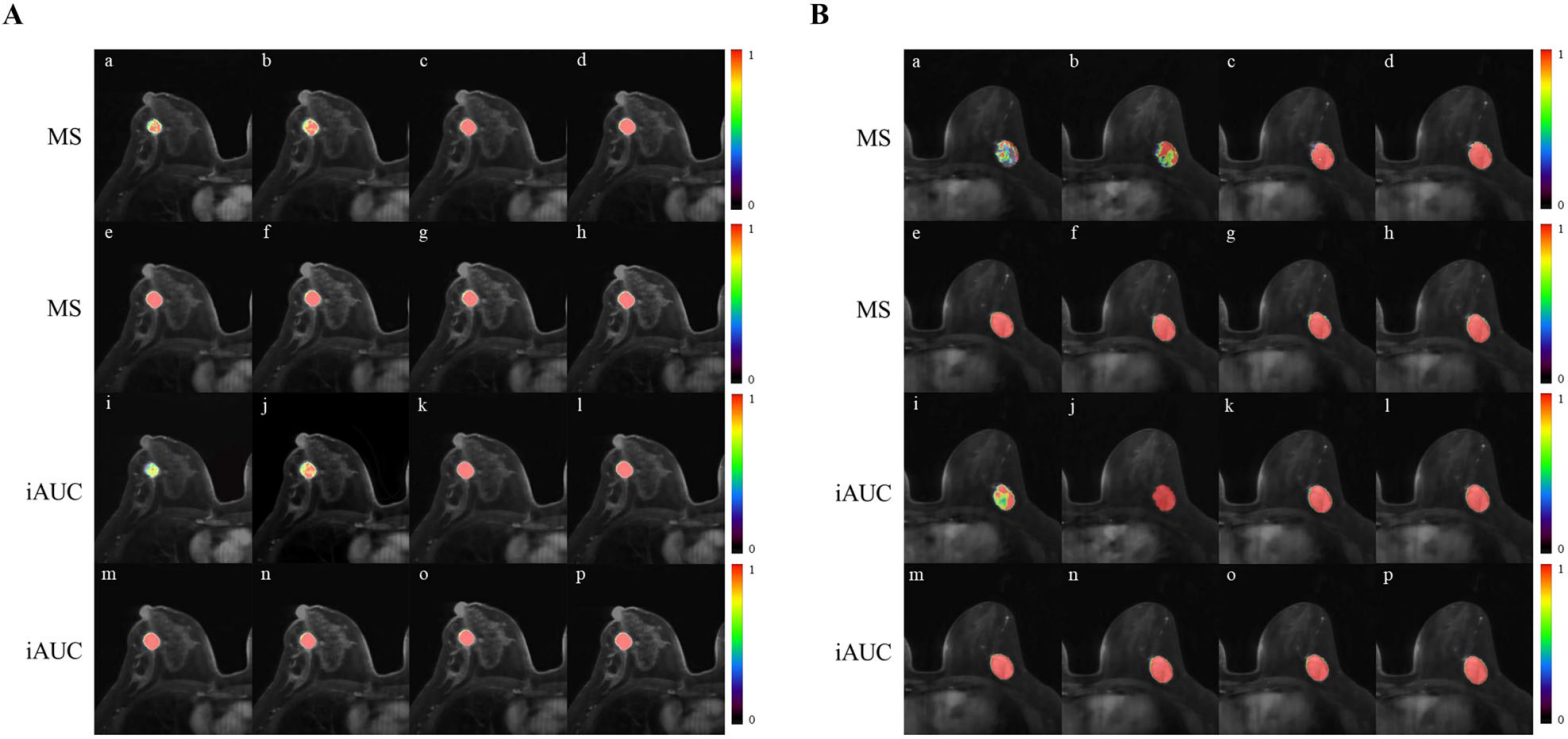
A Ultrafast breast DCE-MRI scans in a 49-year-old woman with pathologically confirmed benign fibroadenoma in the right breast. The first two rows (a–h) show color maps of the MS from SD_40.5s_ to SD_135s_. The last two rows (i–p) show color maps of iAUC from SD_40.5s_ to SD_135s_. B Ultrafast breast DCE-MRI scans in a 53-year-old woman with pathologically confirmed invasive ductal carcinoma in the left breast. The first two rows (a–h) show color maps of the MS from SD_40.5s_ to SD_135s_. The last two rows (i–p) show color maps of the iAUC in 60 s from SD_40.5s_ to SD_135s_. MS maximum slope, iAUC initial area under the curve in 60 s, SD scan duration
